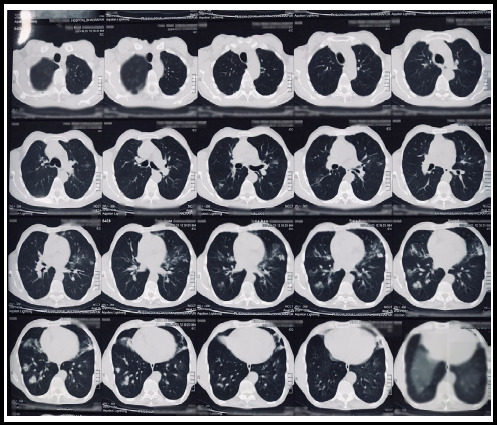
Non-Contrast Computed Tomography (CT) of the chest showing few discrete ground glass nodules in bilateral lungs predominantly right lower and middle lobes and left upper lobe.
